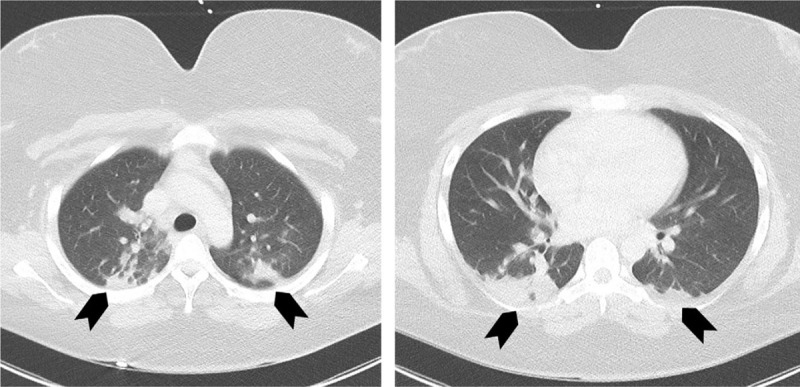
Patient (case 2) chest CT scan. Chest CT scan (axial views) showing typical pneumomia due to SARS-Cov-2 infection with ground glass opacities (arrows).
